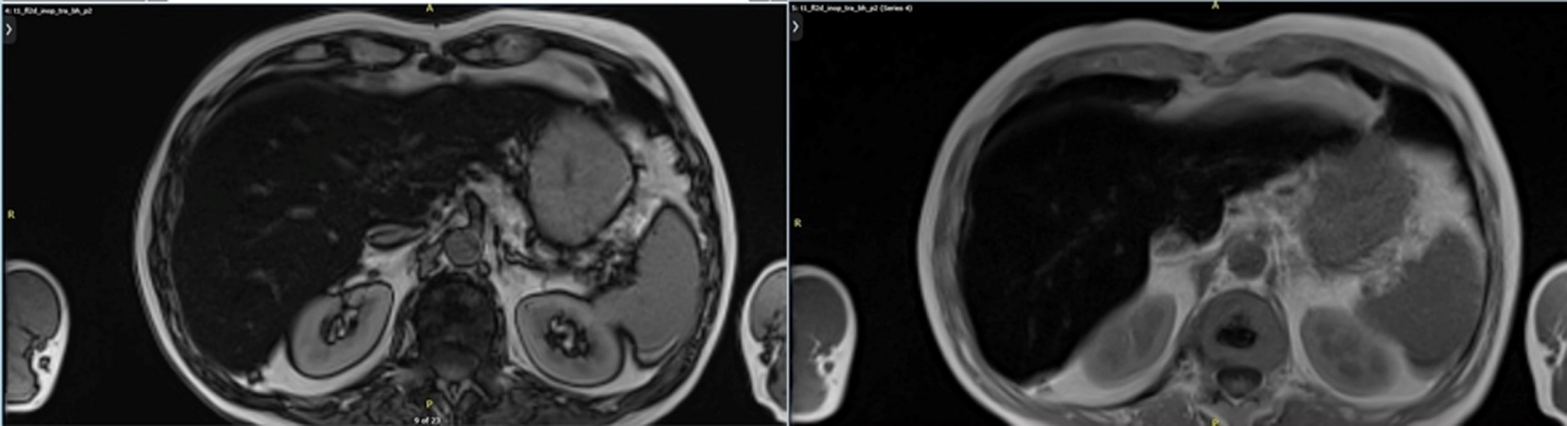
Abdominal MRI showing important hepatic hypointensity and estimating important iron overload at 360 mcmol/gr (VN <36 mcmol/gr).
